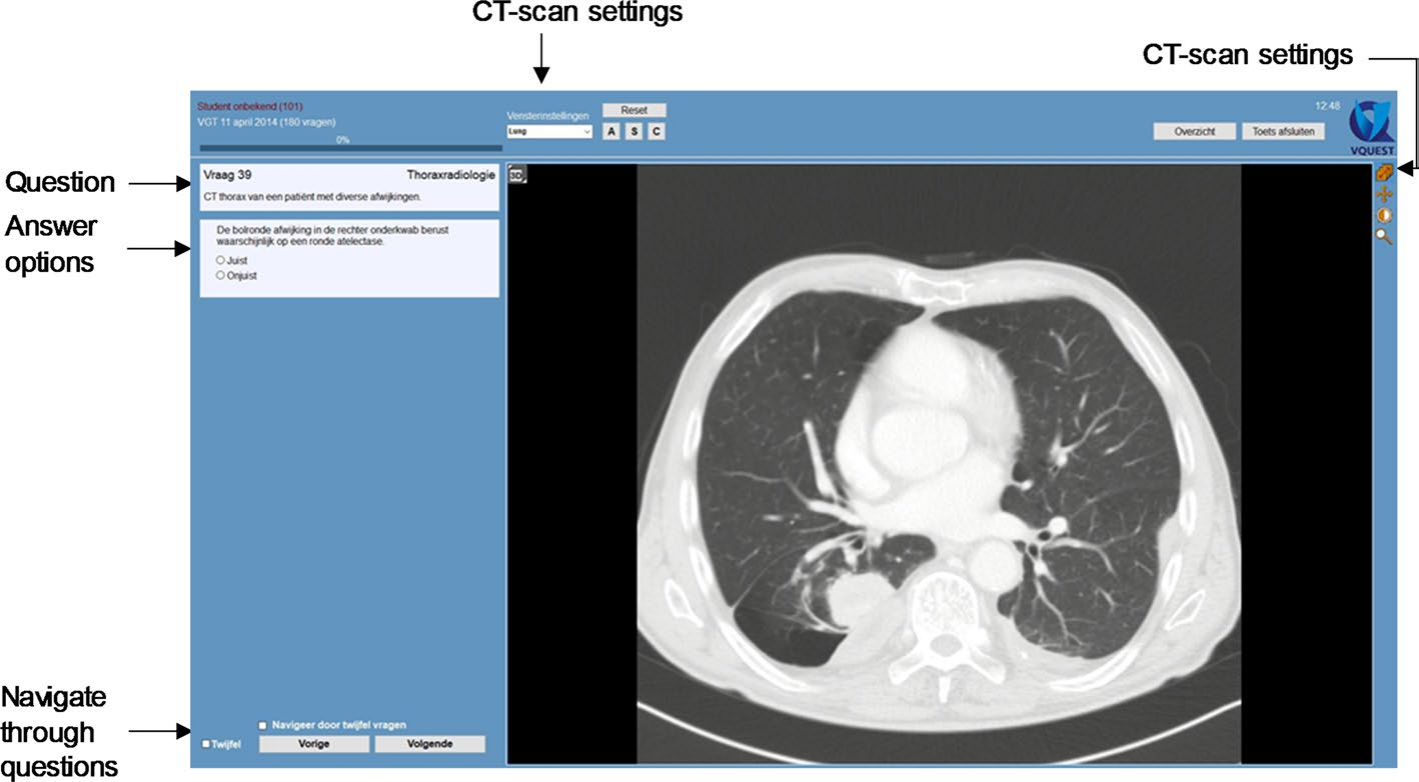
Example of a CT-scan question as displayed to residents in a DRPT
